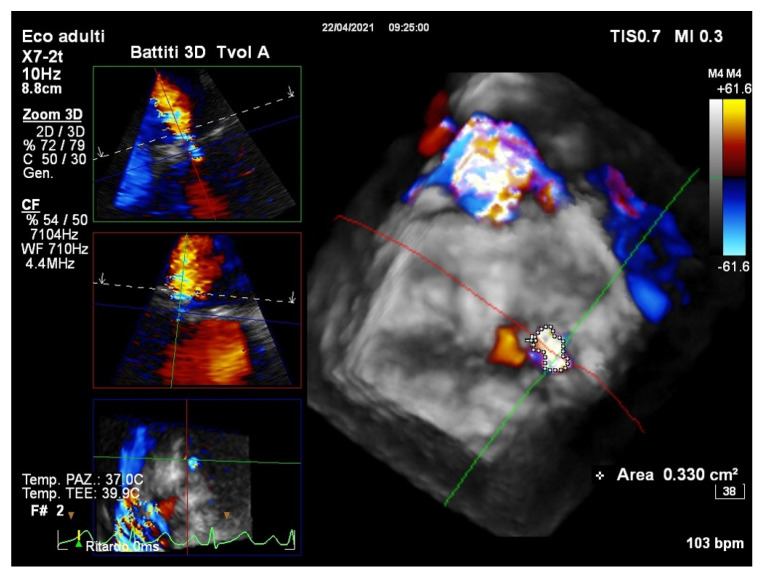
A RT3D color Doppler TEE dataset in a patient with severe AS and functional MR. The display of a 3D analysis software (Philips Medical Systems) showing a 3D view of the mitral valve and the MR jet from an LA perspective (right) and three reconstructed image planes in an orthogonal orientation of the MR jet: long-axis LVOT view (top left), 2-chamber view (middle left), short-axis view (bottom left) showing the asymmetric VCA (0.33 cm^2^ by direct planimetry). Since the formula assumes a relatively round orifice, the PISA by 2D echocardiography tends to underestimate secondary MR. EROA is often highly variable, even when holosystolic, so peak EROA (single frame showing the largest proximal flow convergence zone) can overestimate MR. Several recent studies compared 3D VCA measurements to other methods, especially for the quantification of MR, and found that the more asymmetric the VCA was, the better the accuracy of 3D measurements compared to 2D measurements. However, because of color Doppler blooming effects, the inclusion of low-velocity signals in the tracing, and the orifice’s nonplanarity, 3D VCA can overestimate EROA. Therefore, further clinical research is needed to determine new 3D VCA cutoff values. RT3D: real-time three-dimensional echocardiography; TEE: transesophageal echocardiography; MR: mitral regurgitation; AS: aortic stenosis; LA: left atrial; LVOT: left ventricular outflow tract; VCA: vena contracta area; PISA: proximal isovelocity surface area; 2D: bi-dimensional echocardiography; EROA: effective regurgitant orifice area.
